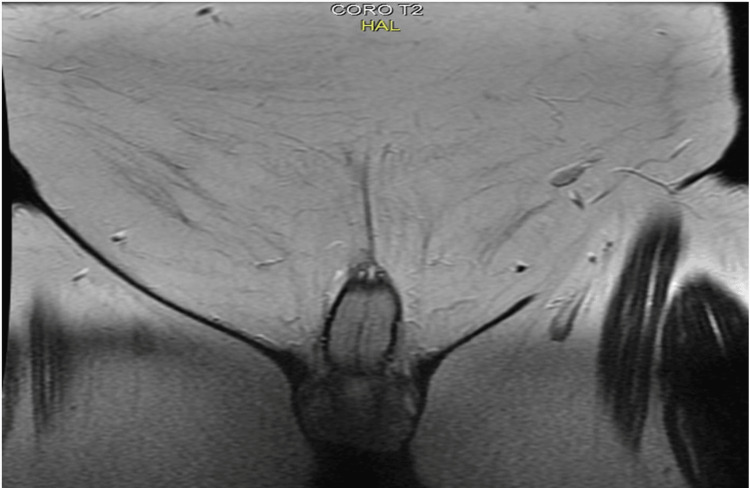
Coronal pelvic MRI T2 showing tumor thickening centered on the glans of the penis, infiltrating the fascia and the spongy urethra with discrete upstream dilatation and bilateral inguinal adenomegaly
